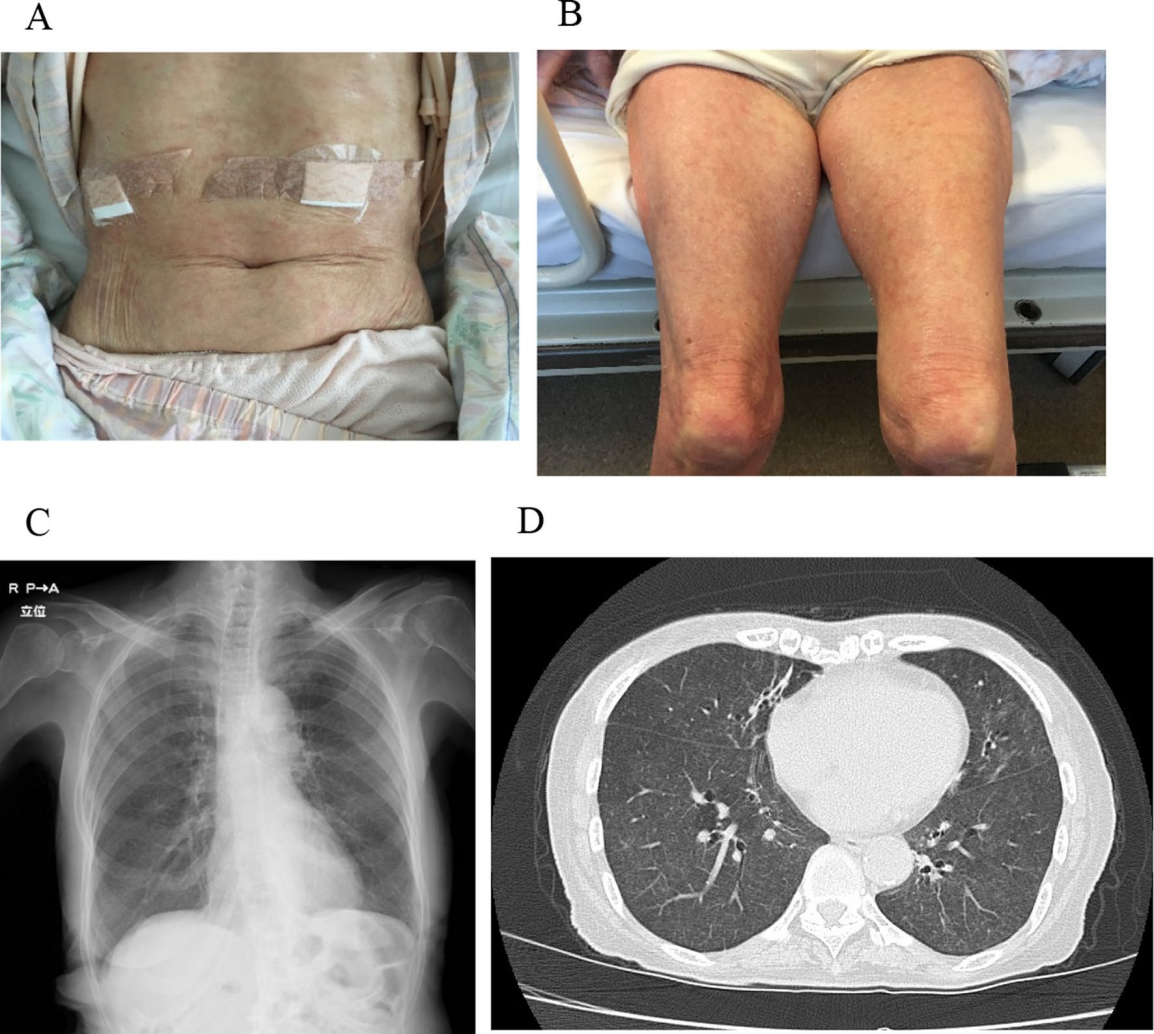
Skin lesions and chest images. A, B Erythematous papules with desquamation are recognized on the trunk and limbs. C Chest X-ray shows bilaterally diffuse granular shadows. D Ground-glass opacities and ill-defined centrilobular nodules are revealed from the central to peripheral regions of the lungs on chest computed tomography
